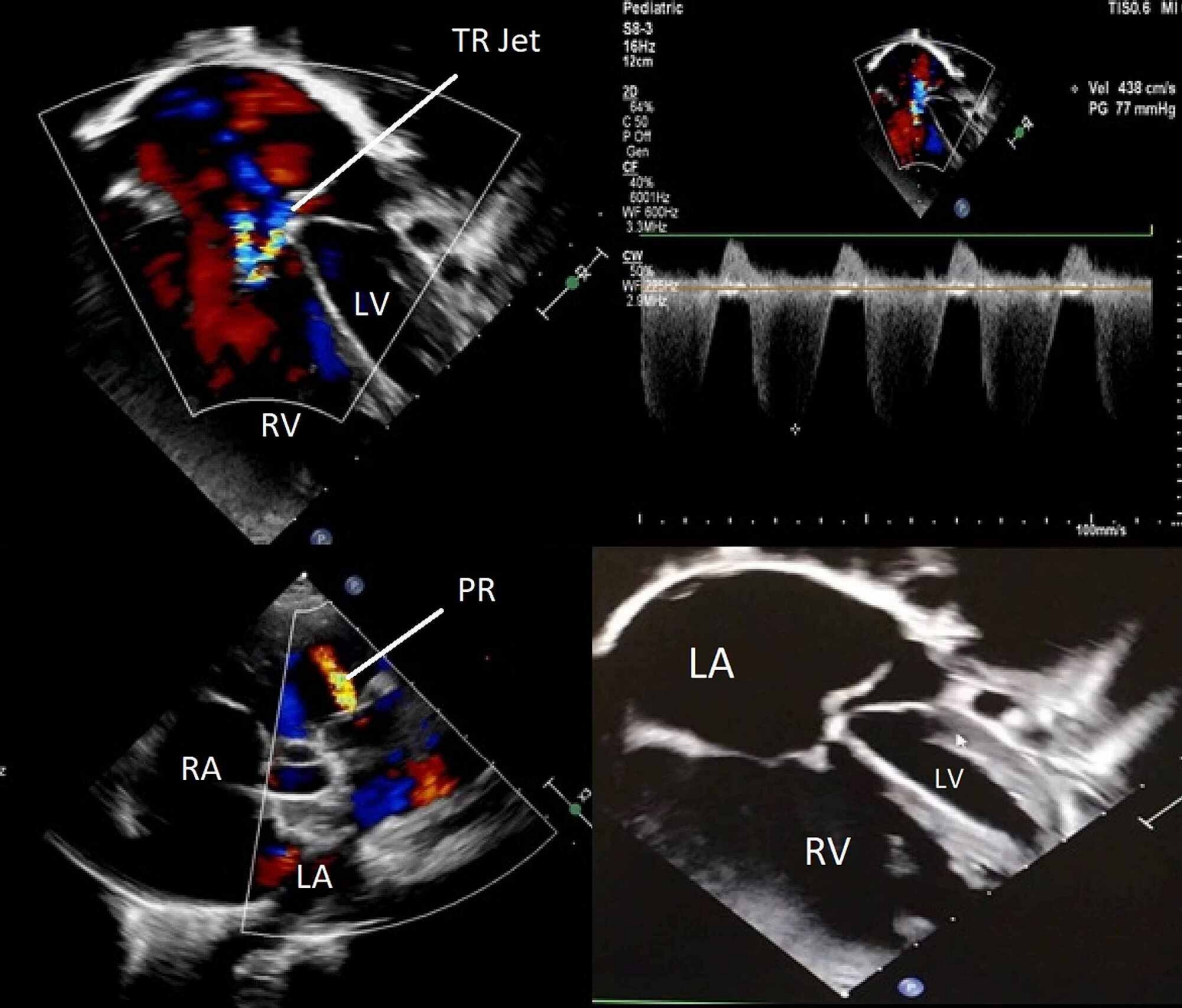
Echocardiography showing dilated IVC with flow reversal, dilated RA, moderate TR with maximum gradient of 77 mmHg. AV: aortic valve; LA: left atrium; LV: left ventricle; PR: pulmonary regurgitation; RA: right atrium; RV: right ventricle; TR: tricuspid regurgitation; TV: tricuspid valve; IVC: inferior vena cava
